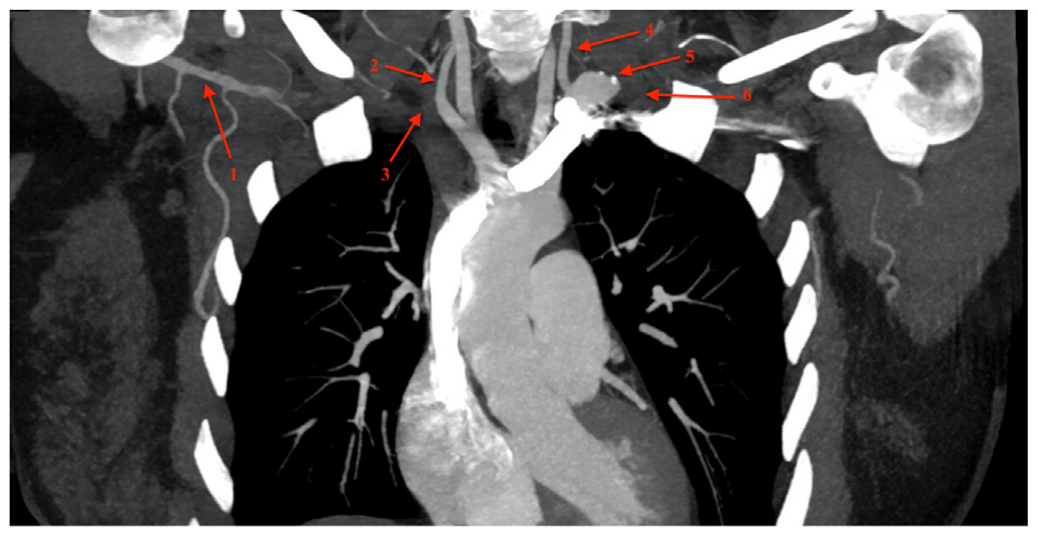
Computed tomography scan showing reconstitution of distal right axillary artery (*1*), right vertebral artery (*2*), expected course of the right subclavian artery (*3*), left vertebral artery (*4*), left subclavian artery aneurysm (*5*), and expected course of left subclavian artery (*6*).
